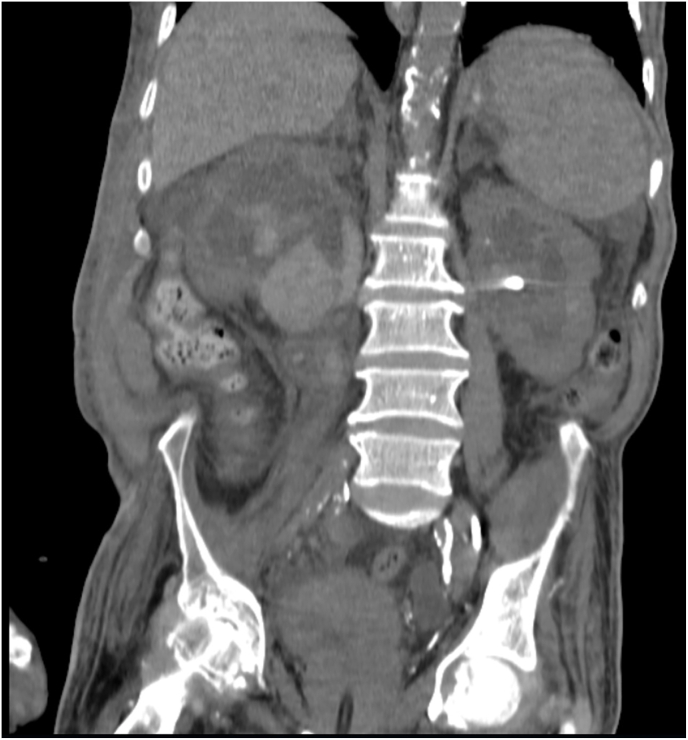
Follow-up CT scan after fibrinolytic therapy and successful clot dissolution, demonstrating marked resolution of the clot burden within the bilateral upper urinary tracts. The hydronephrosis is significantly improved, indicating restored urinary flow. Fig. 2 CT scan of the abdomen and pelvis after the administration of fibrinolytics, showing significant lysis of the clots.
